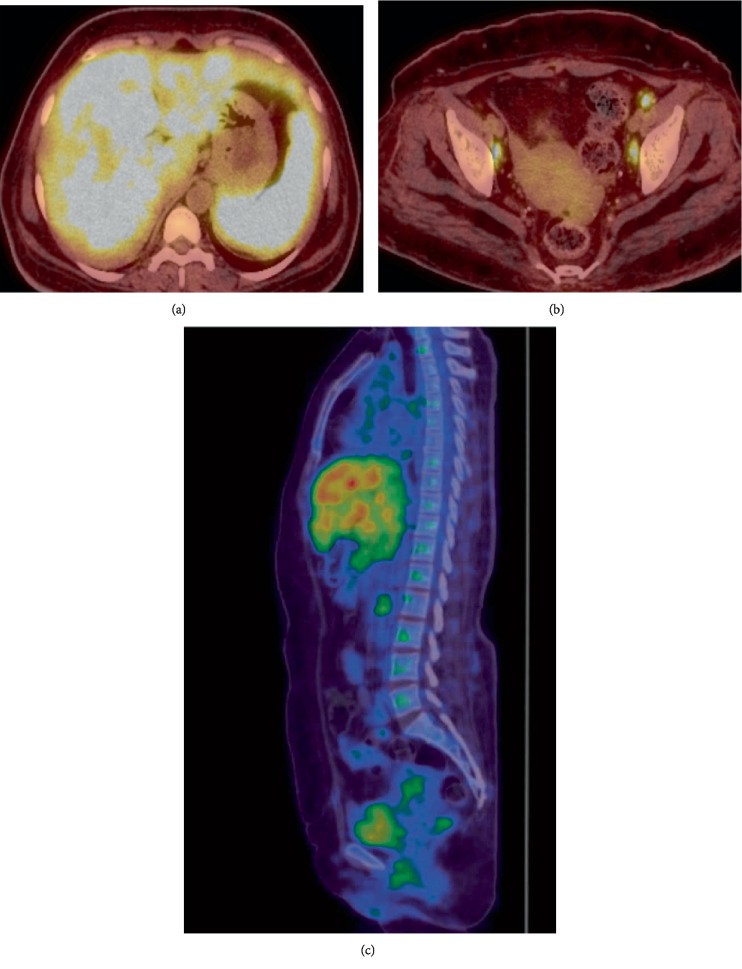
Whole body Flourine-18-fluorodeoxyglucose positron emission tomography/computed tomography (18F-FDG PET/CT). (a) Coronal PET/CT abdomen showing enlarged liver and spleen with multiple ill-defined FDG avid lesions. (b) Coronal PET/CT abdomen showing FDG avidity in few small pelvic lymph nodes. (c) Whole body sagittal PET/CT showing FDG avidity in the liver, spleen and pelvic lymph nodes.
